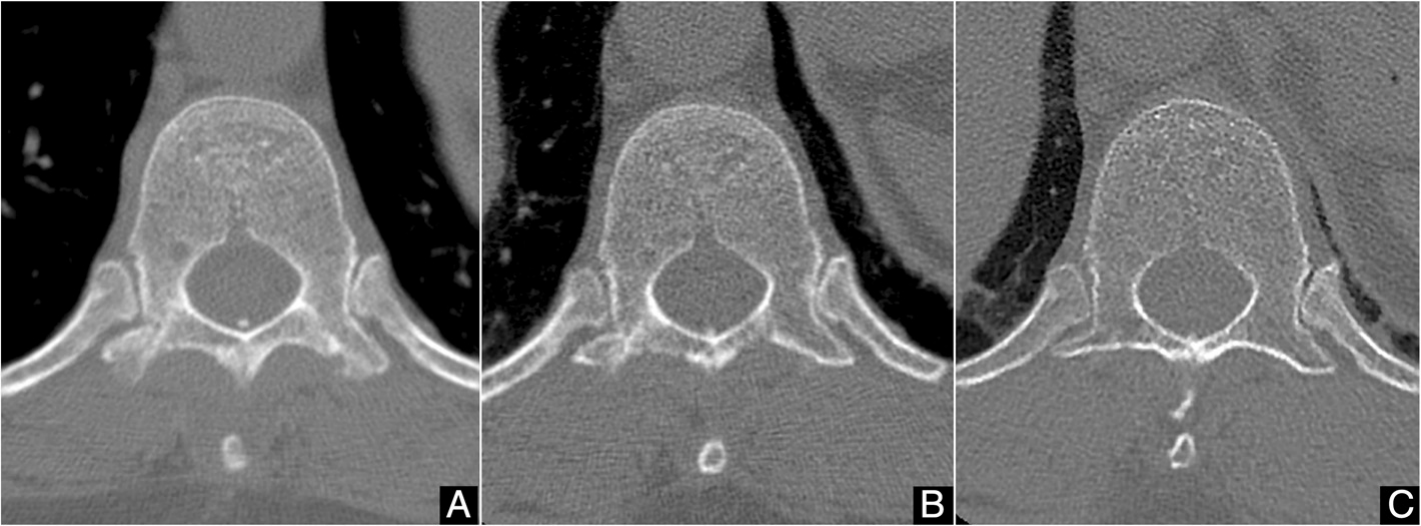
Postoperative computed tomography at the T11 level. a Cut lines between the recapped lamina and host bone at 1 month after surgery. b Bony union is apparent at 6 months after surgery. c Intact spinal canal is confirmed at 5 years after surgery
